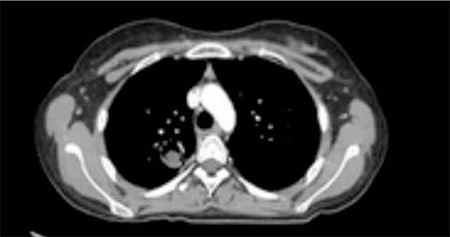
Thorax computed tomography with halo sign.
